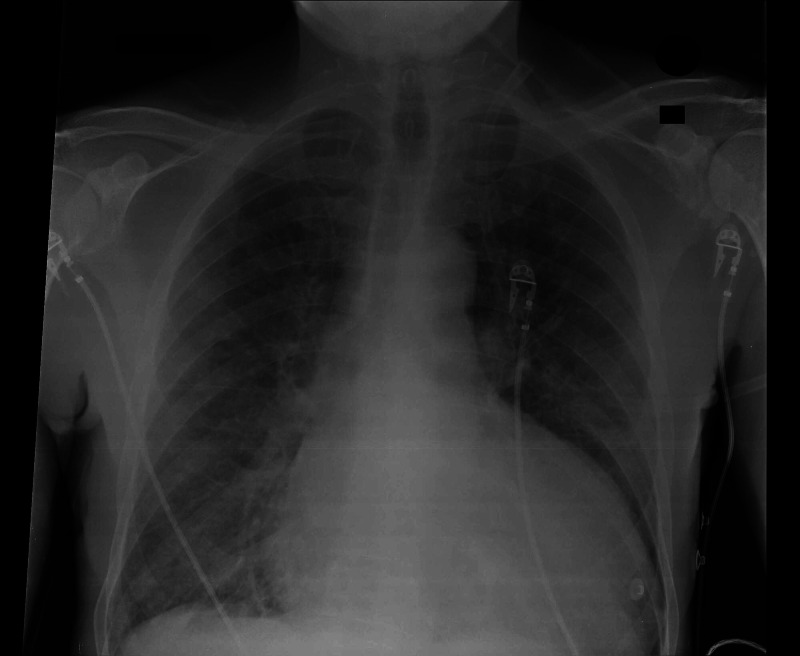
Chest radiograph The chest radiograph showing cardiomegaly and diffuse bilateral pulmonary infiltrates
